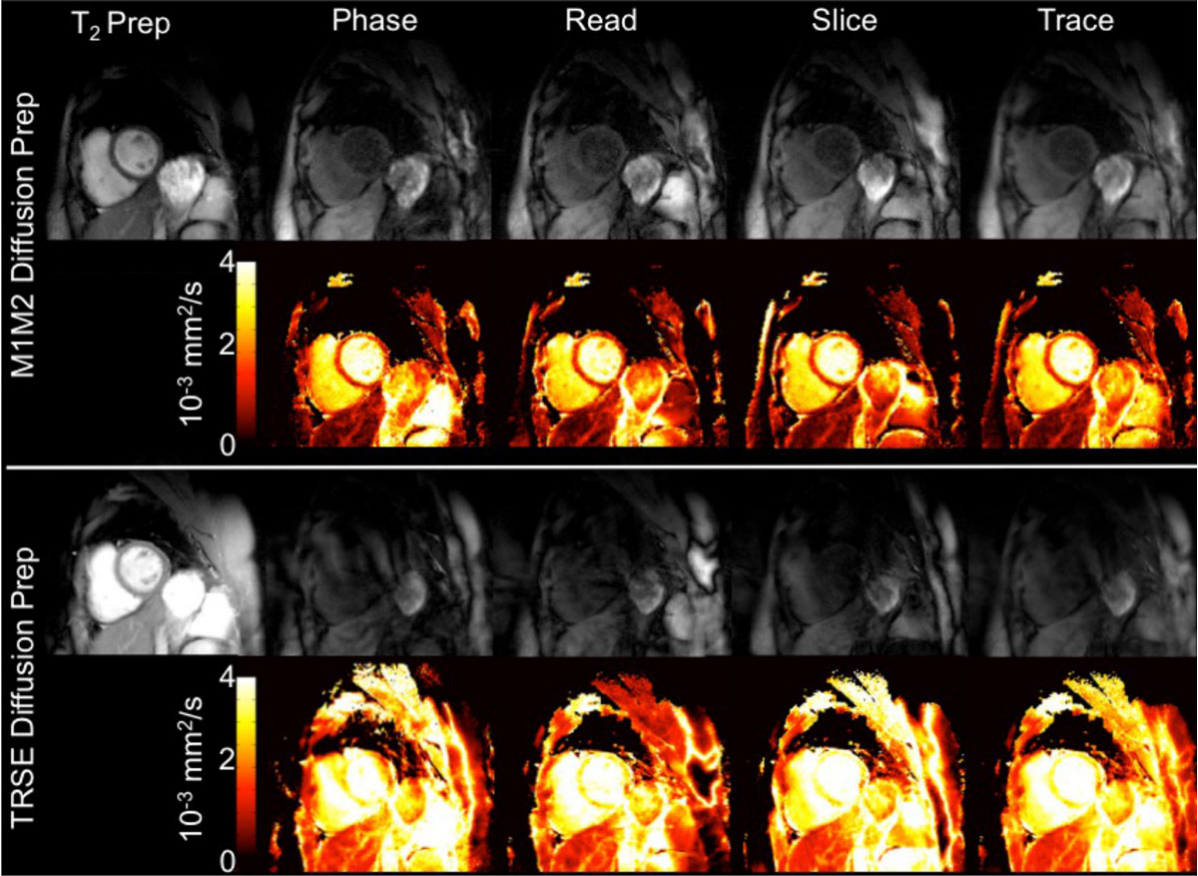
Typical T2 prepared (b = 0 s/mm^2^) images, DWI (b = 450 s/mm^2^) in three orthogonal directions, and ADC maps for M1M2 (top) and TRSE (bottom) diffusion preparation. M1M2 diffusion-prepared images clearly depict myocardium with minimal artifacts and yield reasonable myocardial trADC values. TRSE diffusion-prepared images present ghosting artifacts and signal drop out that are expected to arise in bulk motion corrupted diffusion-prepared sequences. LV trADC value derived from TRSE diffusion preparation are beyond diffusion of free water at 37°C (3.1 × 10^-3 ^mm^2^/s).
